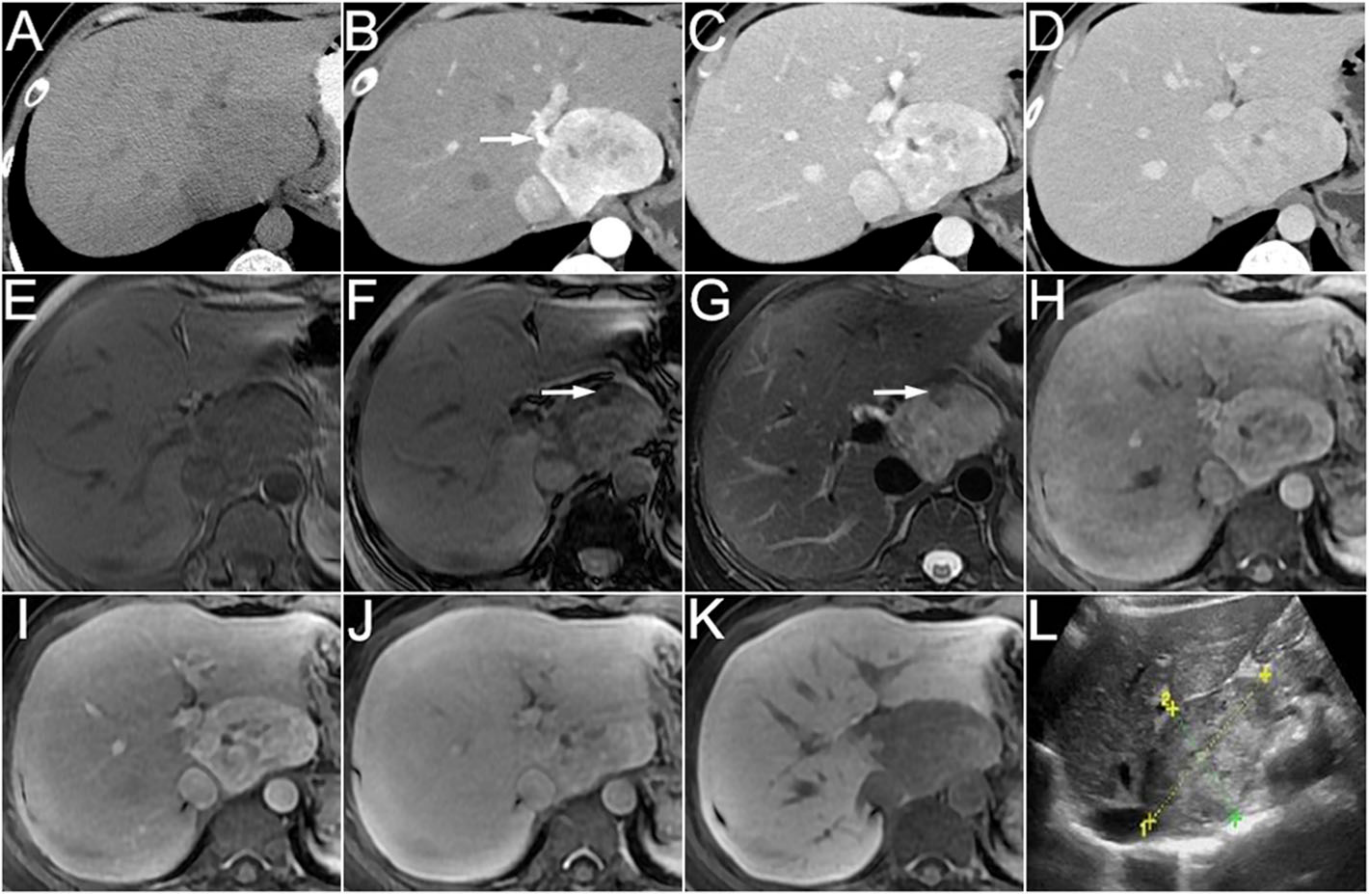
A 35-year woman with hepatic angiomyolipoma. The non-enhanced CT (a) showed a round well-circumscribed homogeneous hypoattenuated mass in the caudate hepatic lobe. On the arterial phase (b), the tumor demonstrated significantly heterogeneous enhanced surrounded by a dysmorphic vessel (arrow). On the portal venous (c) and delayed (d) phases, the enhancement decreased, but still showed higher density compared to the surrounding liver. The MRI (e, T1WI in-phase; f, T1WI out-of-phase; g, fat-suppressed T2WI) images showed hypointense on T1WI and hyperintense on T2WI. Note the fat showed signal intensity decreased on out-of-phase image as compared with in-phase image and low signal intensity on fat-suppressed T2WI (arrow). On hepatocyte-specific agent enhanced MRI (h, arterial phase; i, portal venous phase; j, delayed phase; k, hepatobiliary phase), the tumor showed the same enhancement on arterial, portal venous and delayed phase images as CT and marked hypointensity of the tumor relative to the liver parenchyma on the hepatobiliary phase image. Ultrasound (l) showed a well-defined heteroechoic mass in the liver
